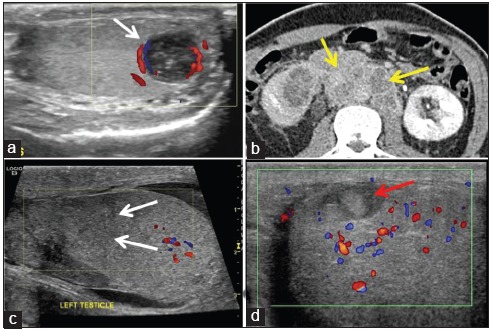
Tuberculosis mimics. A 20-year-old male with scrotal pain. (a) A well-defined heterogeneous hypoechoic lesion in lower pole of testes (white arrow) with peripheral flow on color Doppler. (b) Axial contrast-enhanced computed tomography abdomen of same patient revealed bulky conglomerate lymphadenopathy (yellow arrows) diagnosed as germ cell tumor. (c) A 16-year-old male with trauma. Irregular hypoechoic area in upper pole of testis (white arrows) with minimal hydrocele. (d) A 35-year-old male for follow-up of testicular trauma. Avascular heterogeneous lesion in upper pole of testis (red arrow) diagnosed as hematoma.
